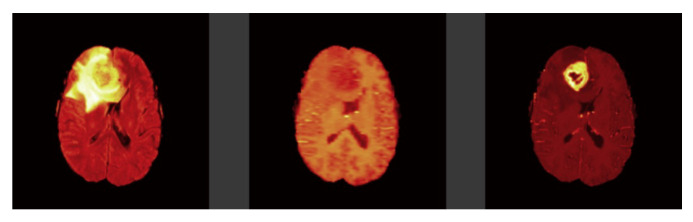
Brain tumor modalities. (Left image: FLAIR, Middle image: T1-weighted, and Right image: T1ce respectively).
